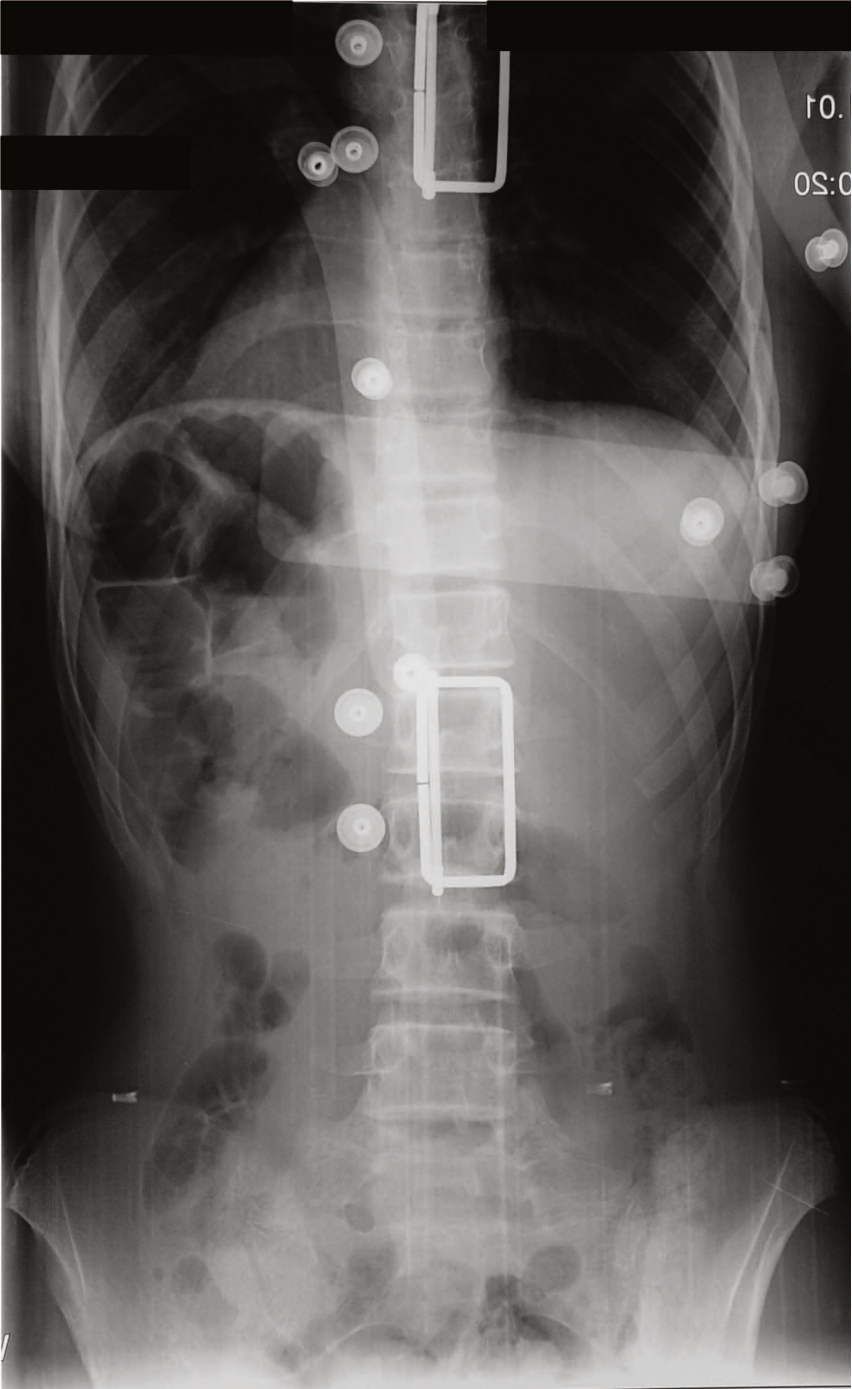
Standing posterior-anterior radiograph of the patient's spine following the application of a modified Boston brace (Visit 2). Notice the immediate derotational effect of the brace.
